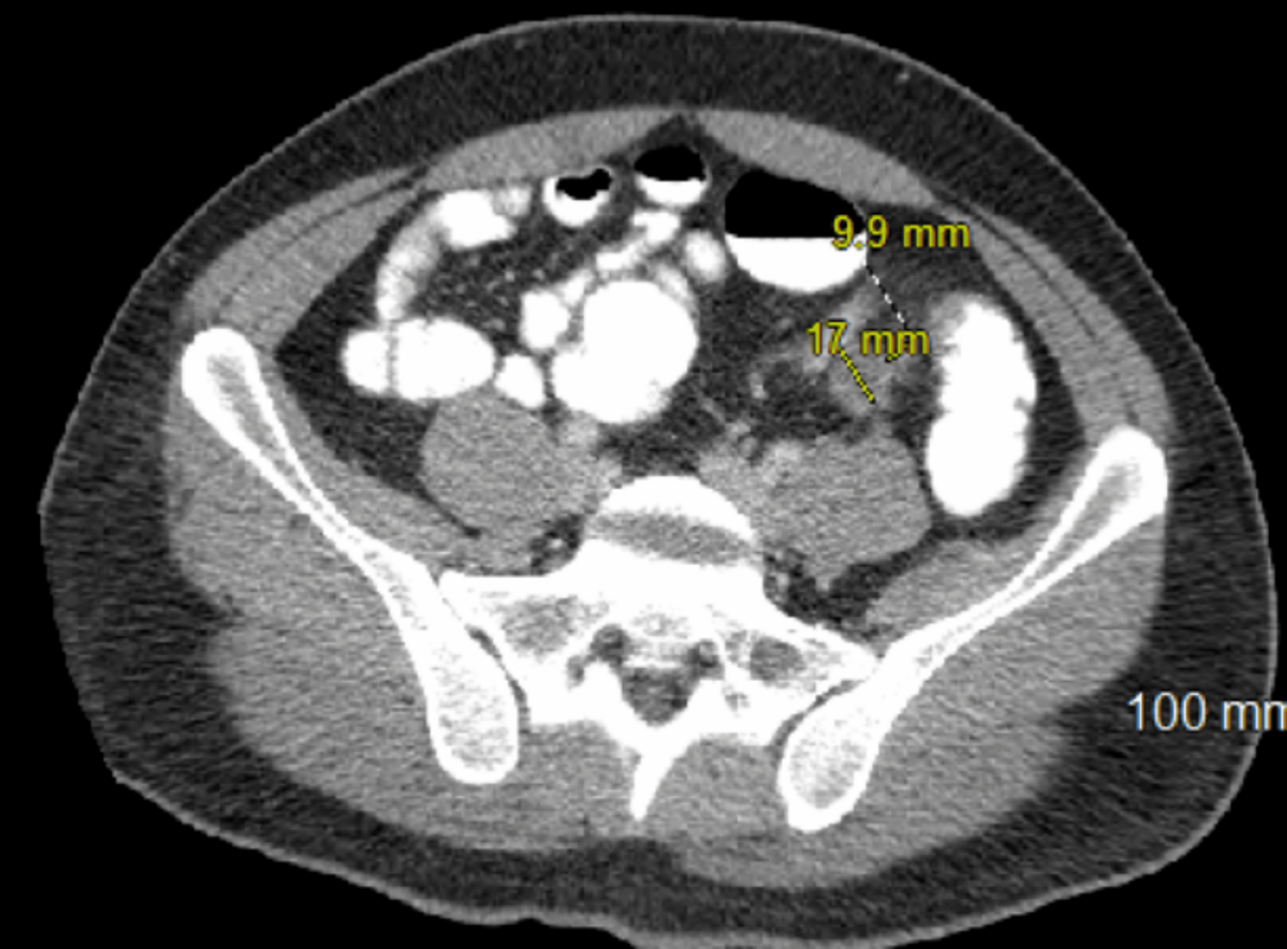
CT abdomen and pelvis (AP) with rectal contrast demonstrating two colonic apple core lesions measuring 1.7 cm and 1.0 cm.
